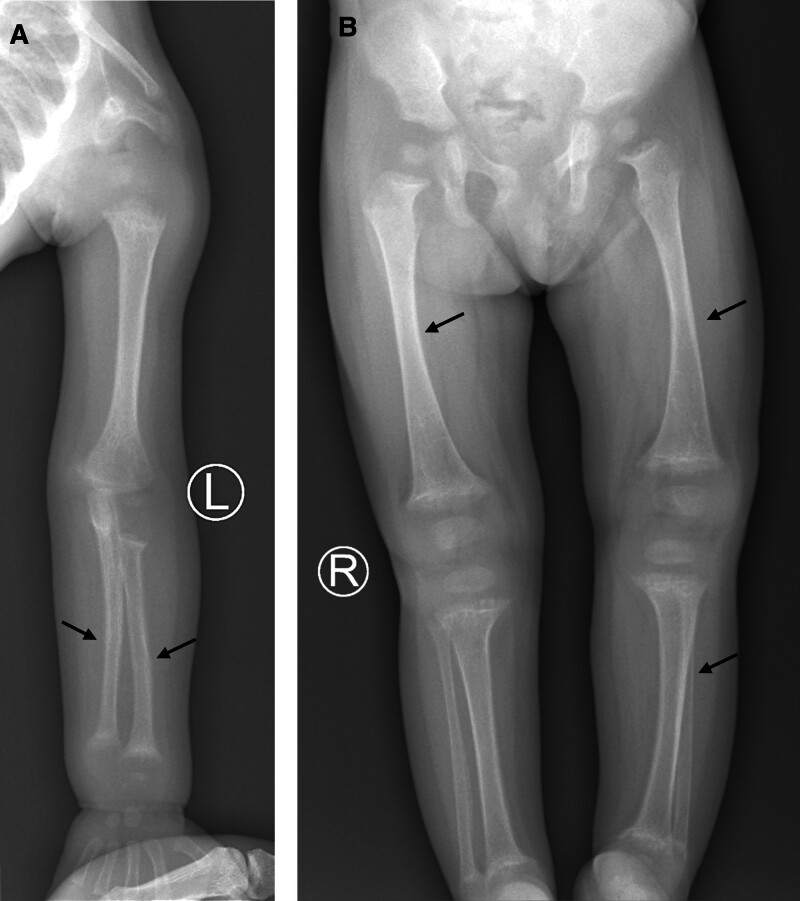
Radiograph assessment of the proband diagnosed with skeletal dysplasia. X-ray images show congenital bowed radius and ulna in the left arm (A), bowed tibia in the left leg, and bowed femur in both legs (B).
